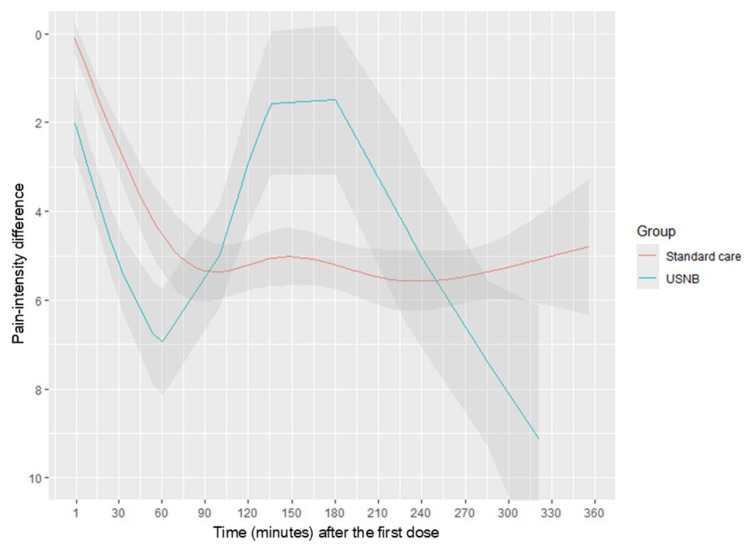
Pain score trajectory over time (minutes) between USNB group and standard group. Line represented the mean of PID and area as 95% confidence interval. PID: pain-intensity difference, USNB: ultrasound-guided nerve block.
